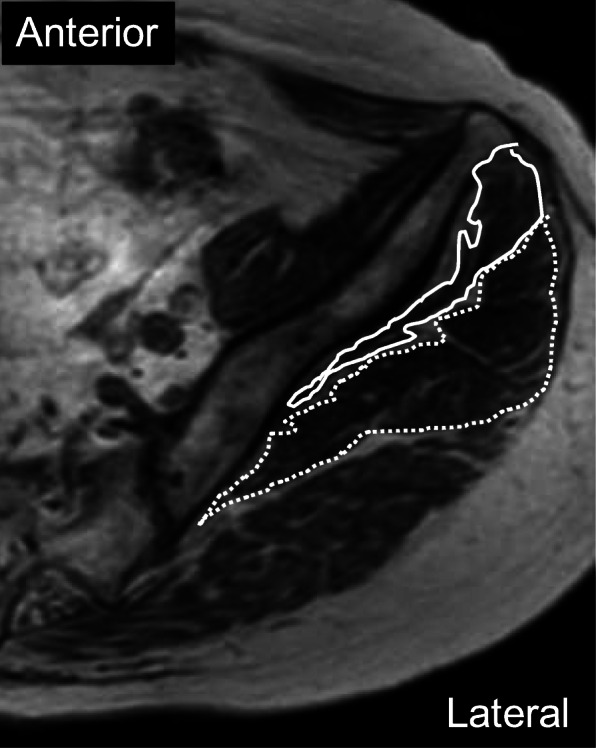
Axial T1-weighted image of the left pelvis at the plane through the bilateral anterior superior iliac spine. CSAs of the gluteus medius (dotted line) and gluteus minimus (solid line) muscles were measured by manually tracing fascia outlines
